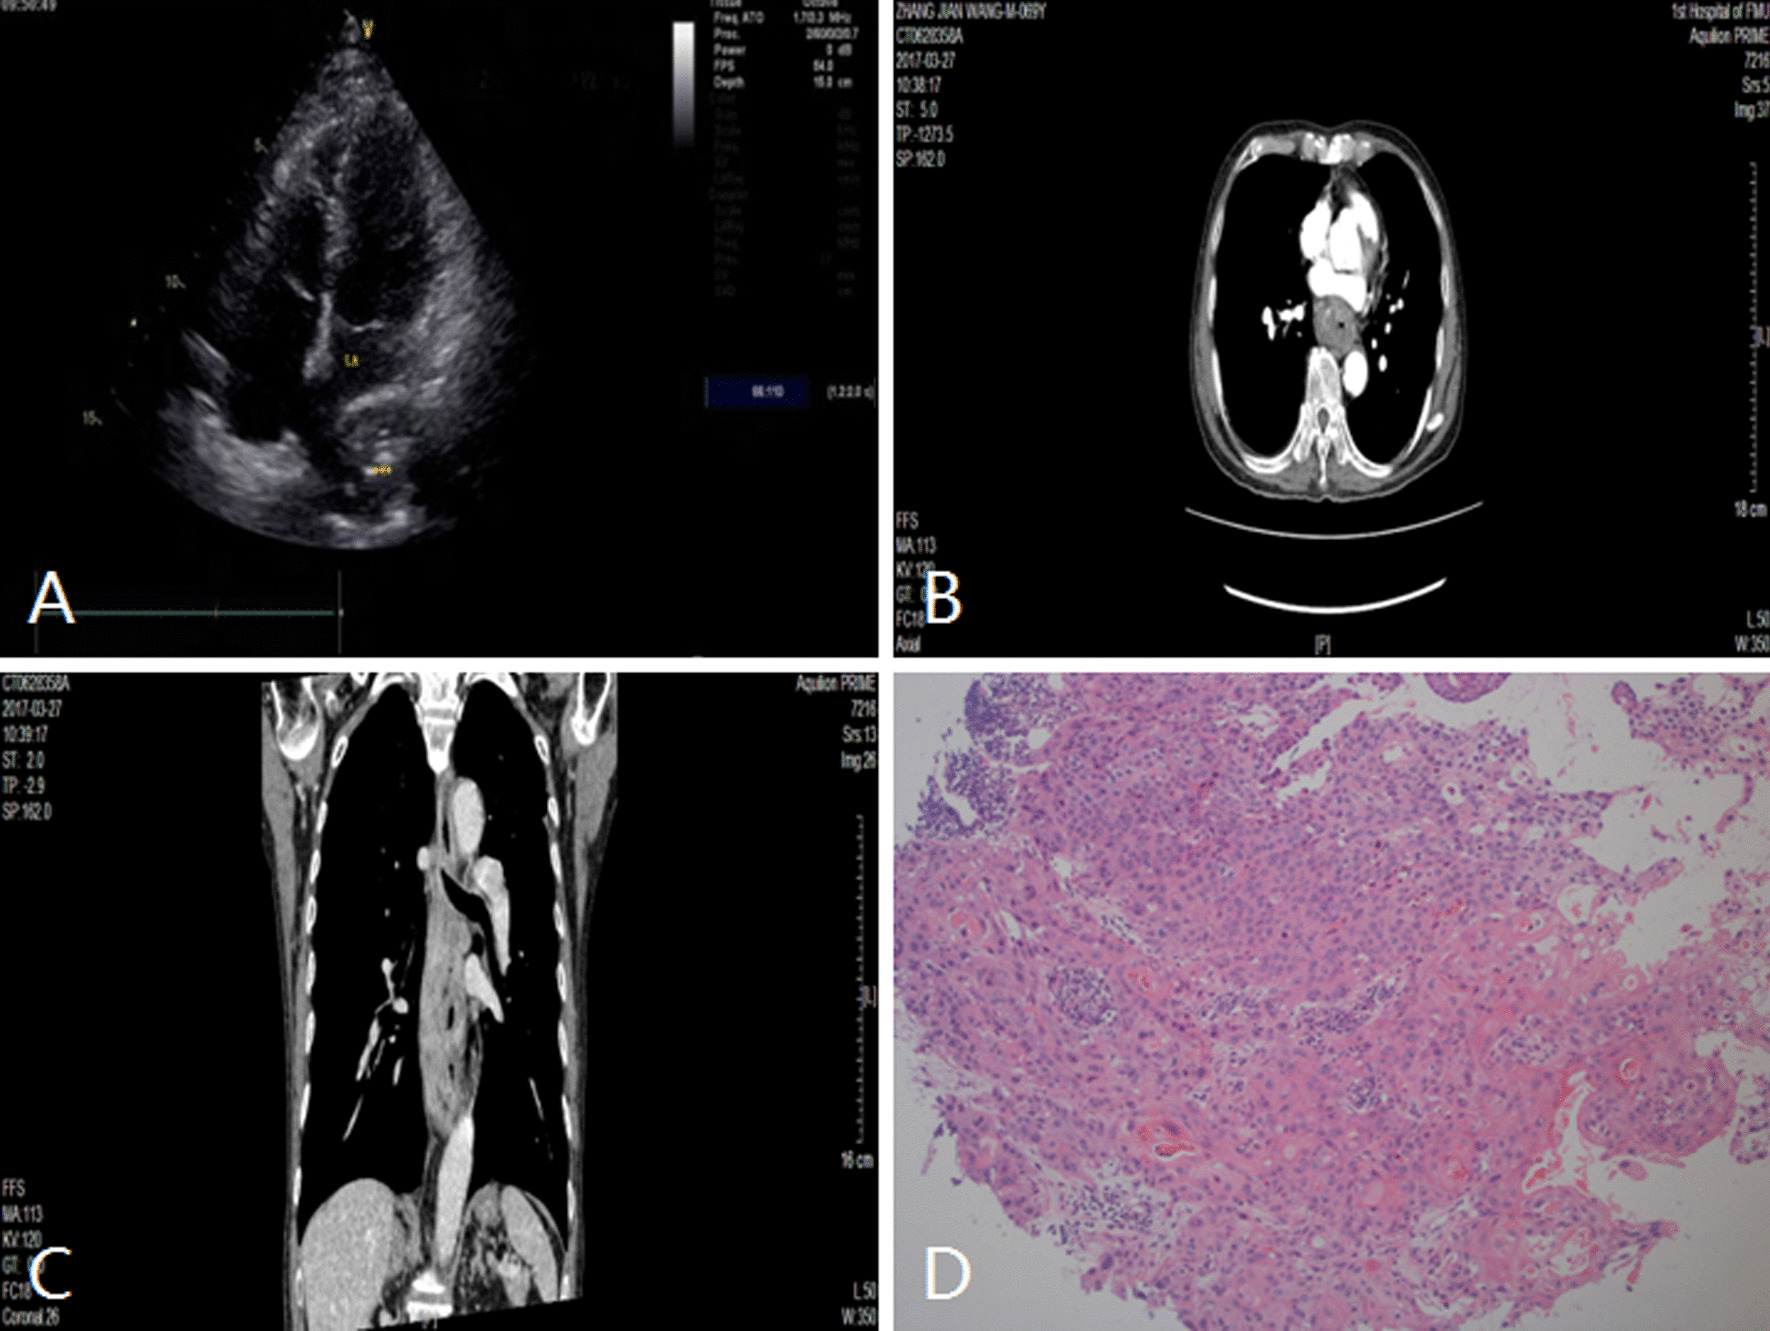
Posterior mediastinal mass (esophageal cancer) in a 69-year-old man. A TTE showed a round hypoechoic or heterogeneous echo mass with hypoechoic periphery and irregular hyperechoic interior, similar to “pseudo kidney” shape. B and C Contrast-enhanced CT showed a regularly shaped mass with inhomogeneous low-density shadow, obvious inhomogeneous enhancement behind left atrium. The esophageal wall was diffusely and irregularly thickened, and the corresponding lumen was irregularly narrow. D The pathologic diagnosis of this mass was esophageal squamous cell carcinoma. TTE indicates transthoracic echocardiography; asterisks, mass; LA, left atrium
